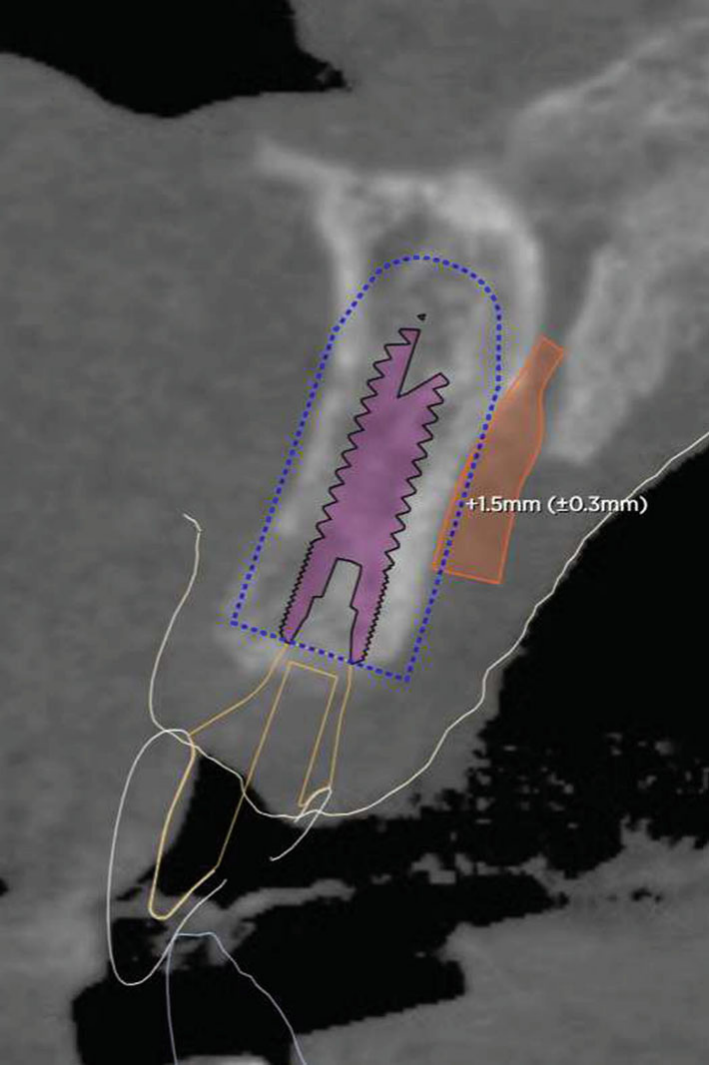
Sagittal radiographic view of implant positioning.
